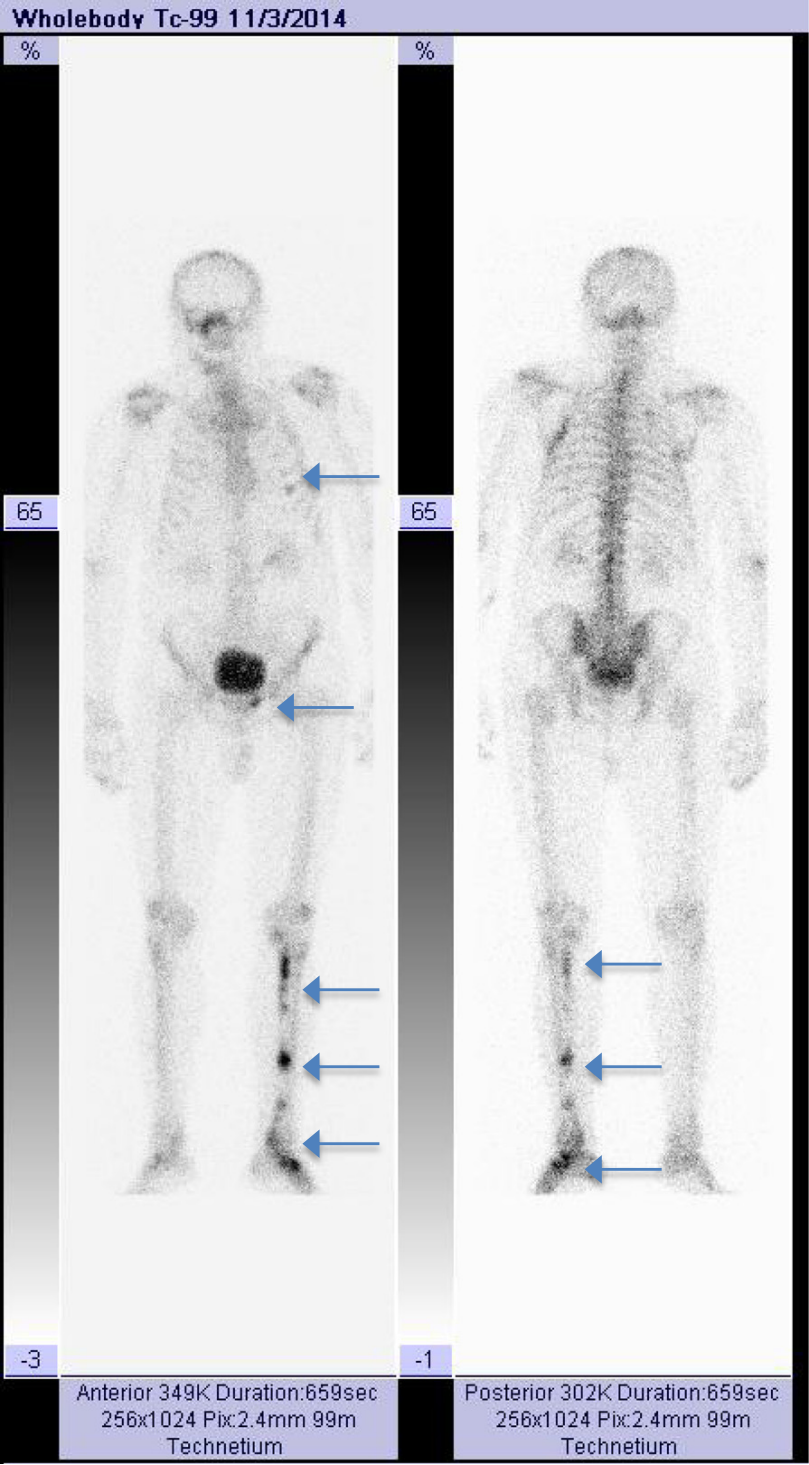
Whole body bone scintigraphy with Tc-99m MDP at 3 years after the initial diagnosis, showing pathologic uptake of the tracer in thorax and left tibia, suggesting evolution of the disease in the left leg (indicated by arrows)
